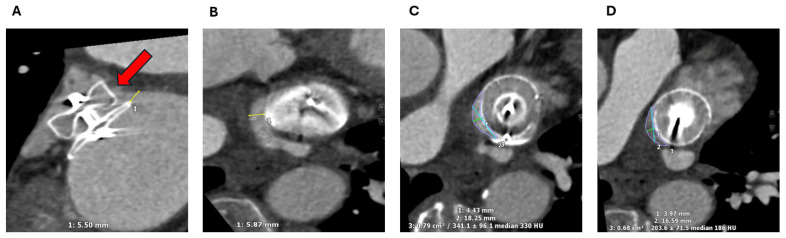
A 73-year-old woman post LAAO with an Amplatzer Amulet device. Multiplanar cardiac CT images show a clear antero-superior PDL tract between the LA and the distal LAA (red arrow) (A). The tract measures up to 6 mm at the level of the disc (A,B). At the mid-lobe level (C), the tract measures 4 × 18 mm with a cross-sectional of area of 0.79 cm^2^. At the distal edge of the lobe (D) it measures 4 × 17 mm with a cross-sectional of area of 0.68 cm^2^. The Hounsfield Units in the distal LAA measures 276 HU, compared with the LA of 336 HU (i.e., more than 25% and 100 HU). LA: Left atrium; LAA: Left atrial appendage; LAAO: Left atrial appendage occlusion; CT: Computed tomography; PDL: Peri-device leak; HU: Hounsfield Units.
